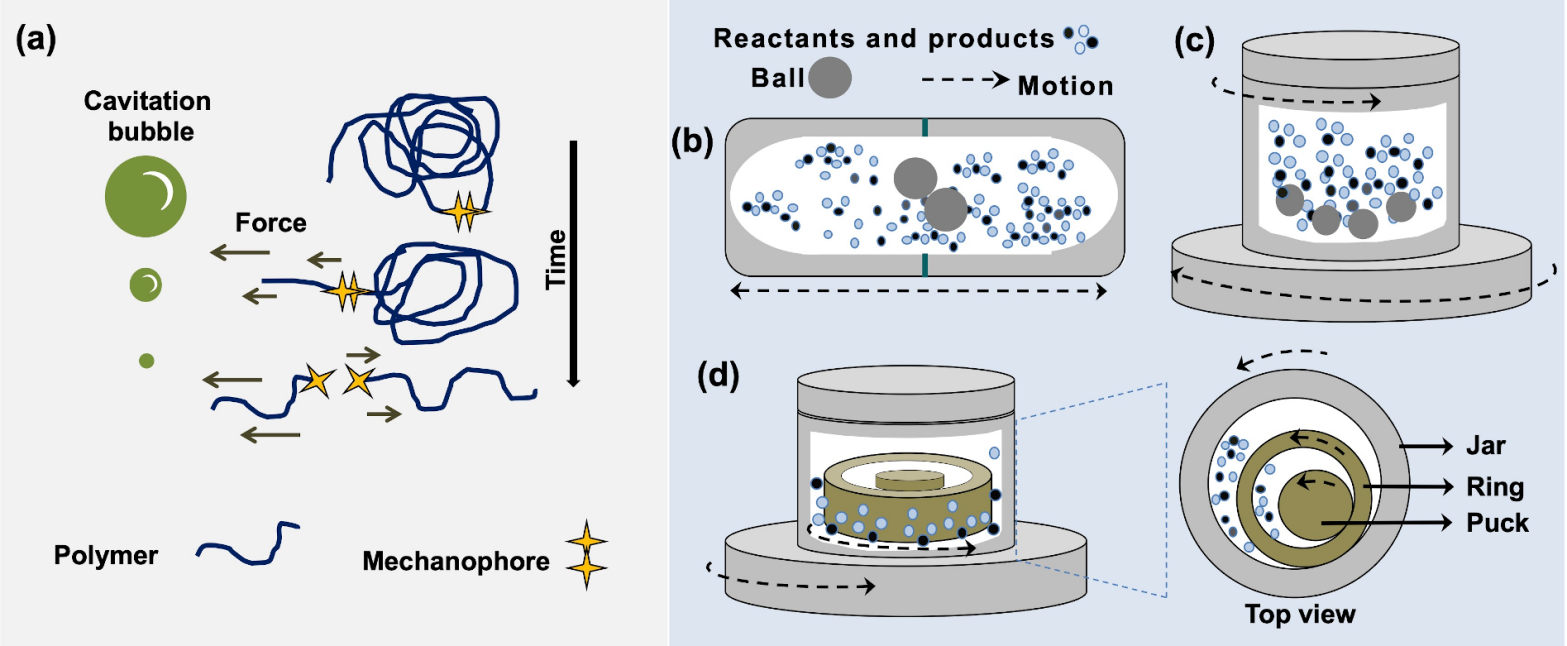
Representation of (a) cavitation and elongational flow caused by pulsed ultrasonication, (b) mixer mill, (c) planetary ball mill, and (d) ring-and-puck mill (vibrating disc mill).
